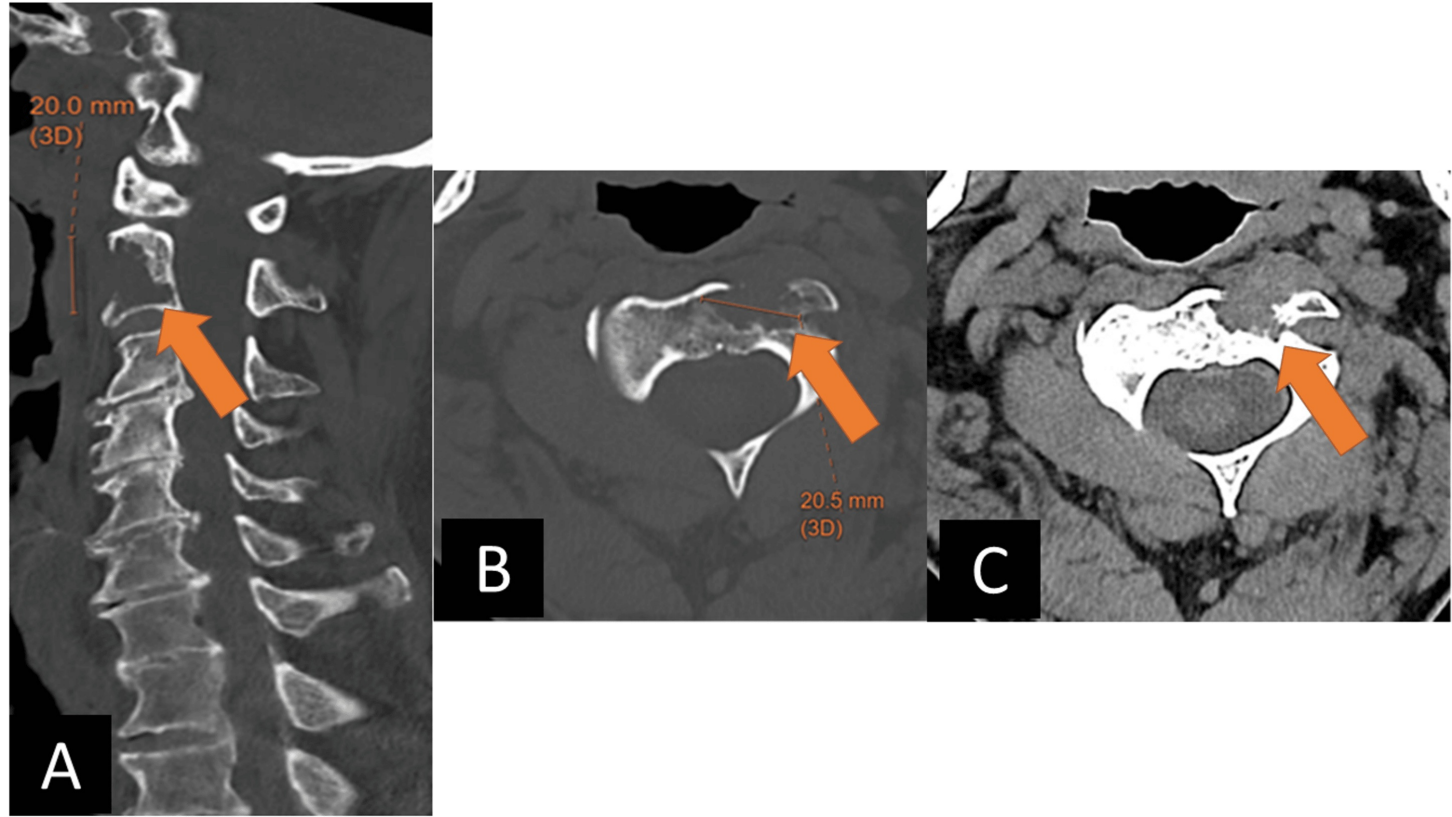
Repeat imaging of the cervical spine shows enlargement of the ventral C2 body lesion with progressed anterior cortical destruction, new involvement of the left foramen transversarium, and subtle extraosseous soft tissue anteriorly. A) sagittal bone window CT; B) axial bone window CT; C) axial soft tissue window CT.
